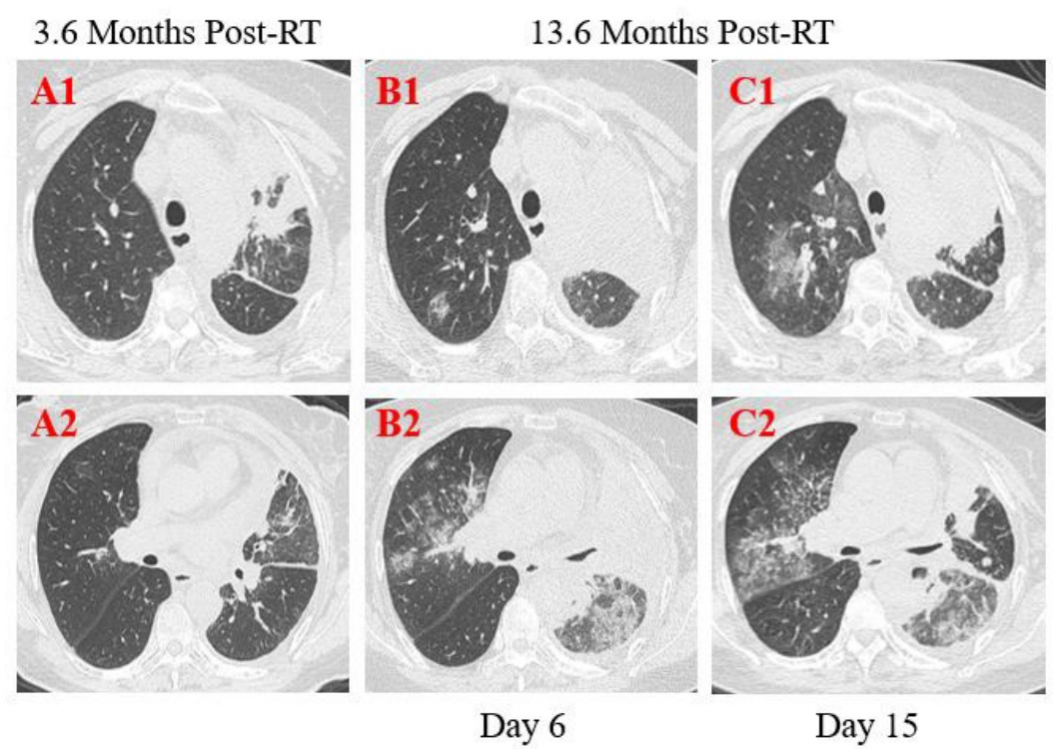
Transverse thin-section serial CT scans from a 55-year-old female with suspected COVID-19 pneumonia. Chest CT images performed on the 6th day after symptom onset indicated an enlarged mass with calcification in the left upper lobe and lung hilum and multiple mediastinal lymph node metastases (A1, B1, C1), bilateral diffused ground-glass opacities with partial consolidation (B2). Follow-up CT on the 15th day demonstrated continuous development in the scope and extent of lung lesions (C1, C2).
